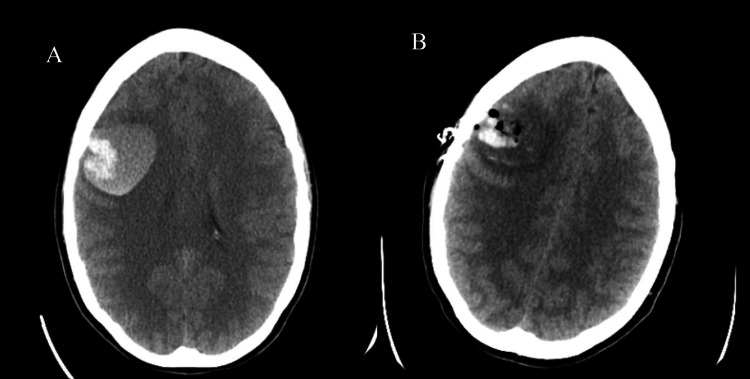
(A) Initial brain CT without contrast shows parenchymal hemorrhage in the right frontal lobe. (B) Post-operative brain CT without contrast shows a decrease in the size of hemorrhage and edema, with minimal left midline shift.
